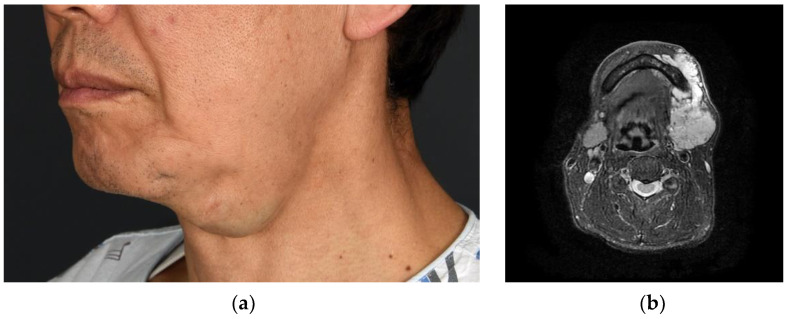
VM in the left submandibular area. (a) Bulging blueish vascular mass was observed; (b) MRI findings of VM. These T2-weighted with fat suppression MRI findings showed an infiltrative vascular channel with a size of 8.2 × 4.1 cm from the subcutaneous fat layer to the submandibular gland and part of the posterior belly of the digastric muscle in the left submandibular area. It showed inhomogeneous patchy high signal intensity inside. VM, venous malformation; MRI, magnetic resonance imaging.
